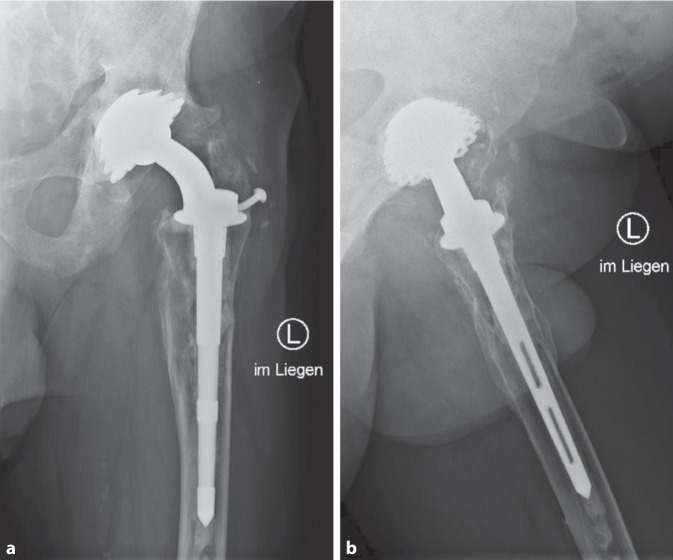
Anterior-posterior (a) and Lauenstein view (b) radiographs of the presented case (left hip). The left hip shows some periarticular ossifications. A screw that was inserted in the collar of the prosthesis has loosened over the years but appears to be locked in its current position. The patient has no complaints here. It is unclear whether some kind of cement was used during implantation of the prosthesis or whether the dense areas within the bone originate from the first operation
